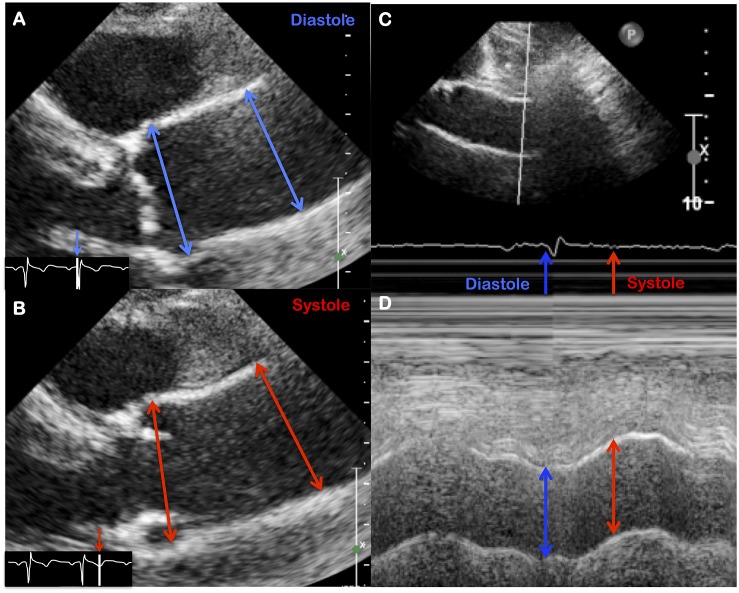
Evaluation of aortic strain using echocardiographic features, either with B mode (A,B) or with M mode (C,D).
